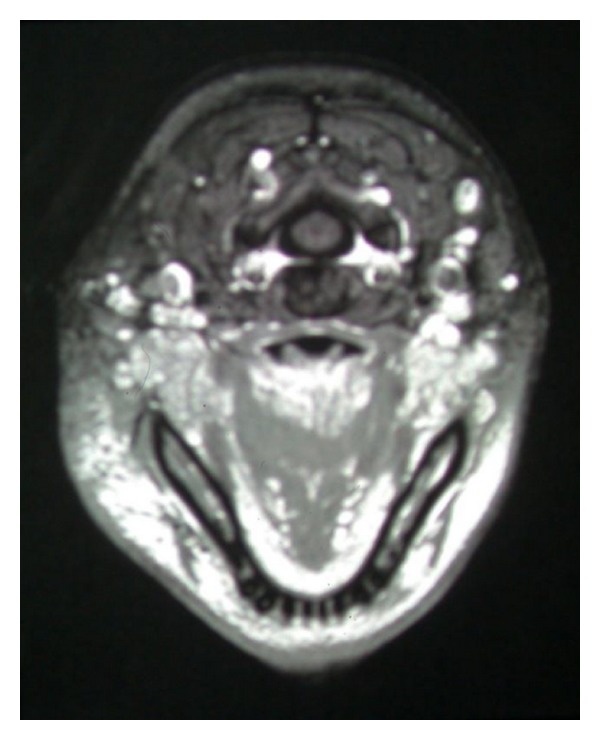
CT of the left parotid mass.
